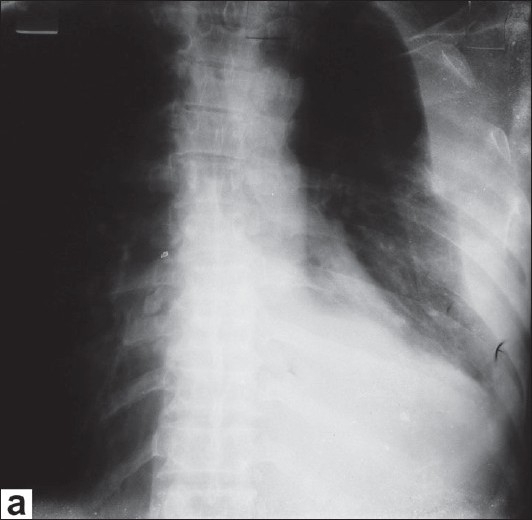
Radiograph chest posteroanterior view
